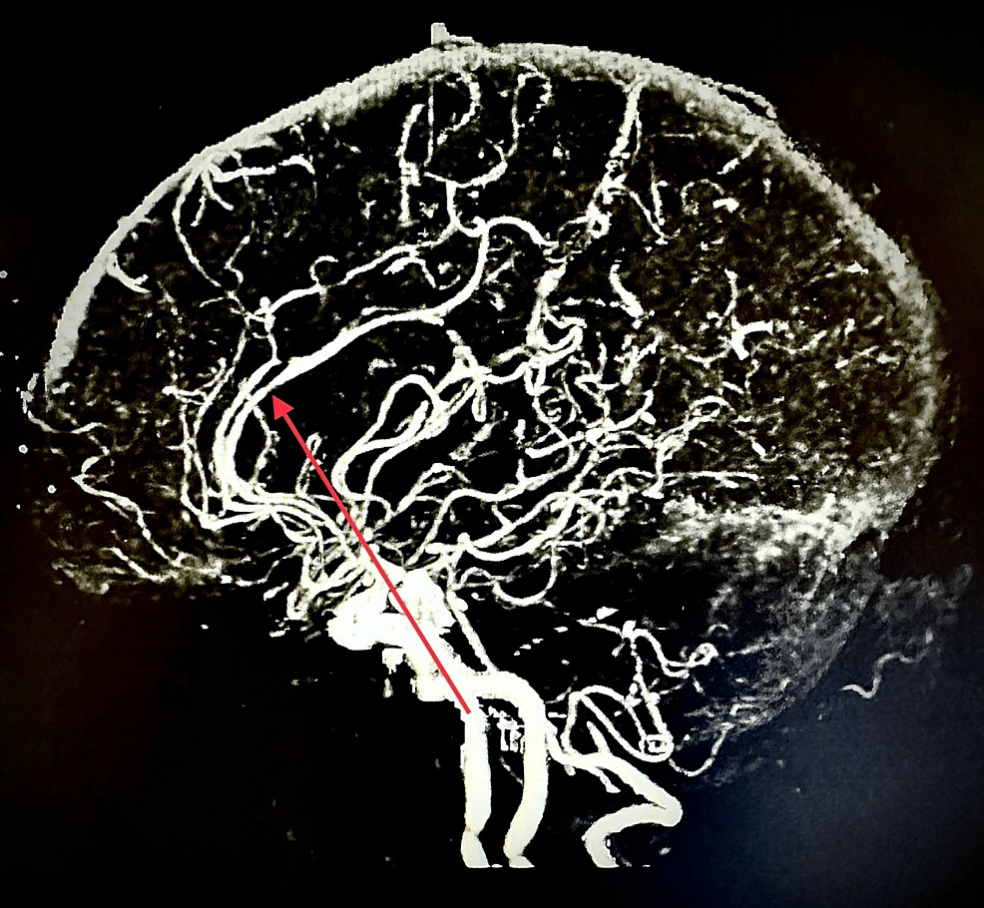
CT angiogram of the head and neck with and without contrast showing focal narrowing (approximately 50%) of the left M2 segment. CT, computed tomography
